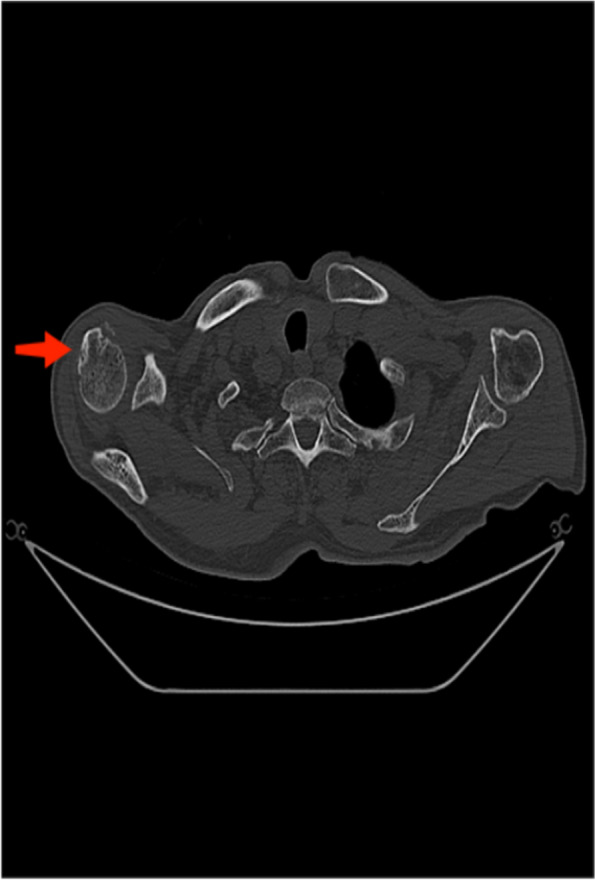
Plain CT scan of the humerus. Male, 80 years old
